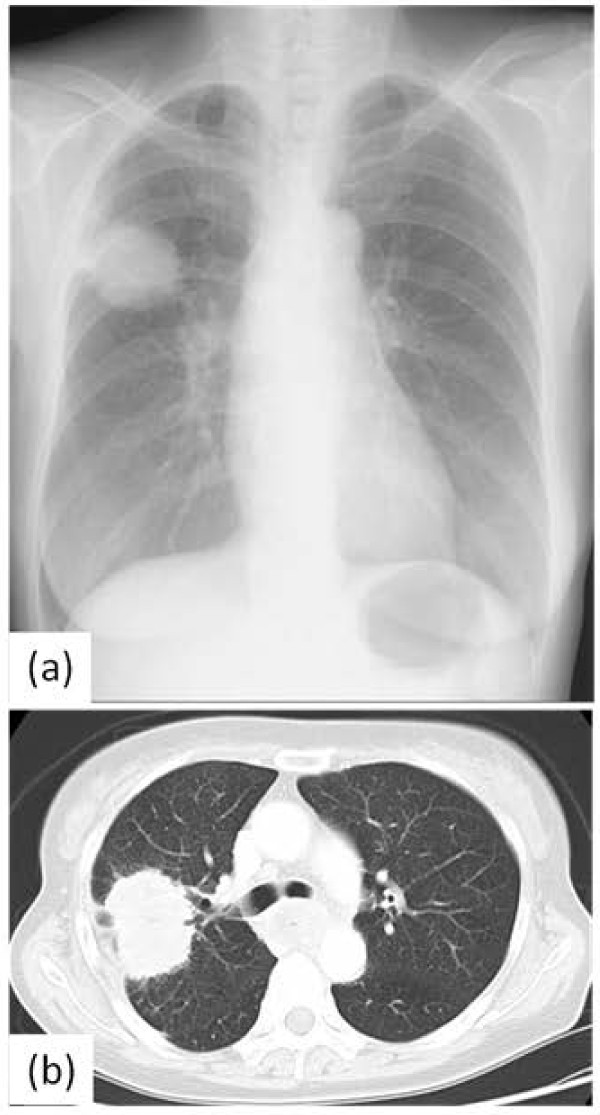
Chest X-ray and computed tomography(CT) shows a huge tumor in the right lung field. (a) Chest X-ray shows a 55 mm round mass in the right upper lung field. (b) Chest computed tomography scan reveals the mass in the right upper lobe with infiltration to B2 and B3a bronchus.
